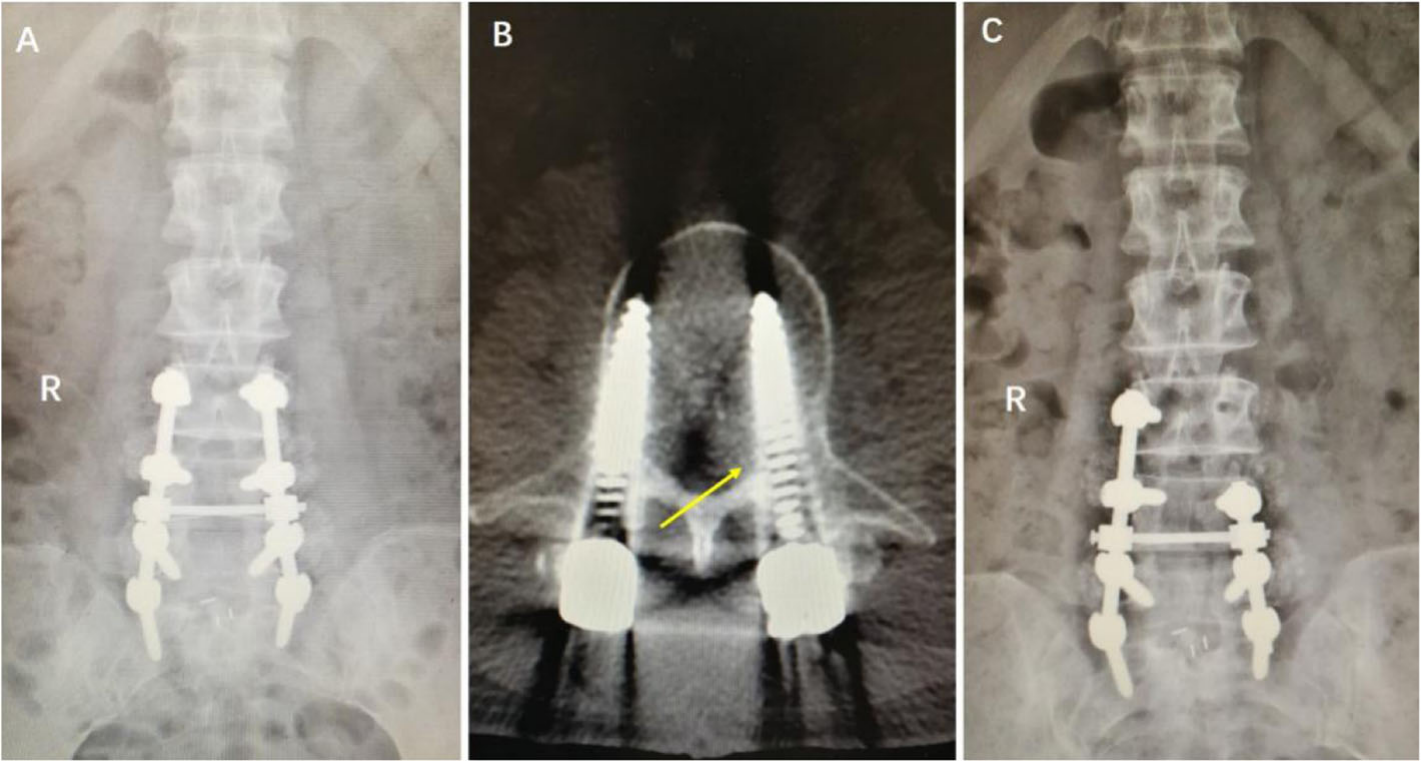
A 62-year-old female experienced pain in the front of her left thigh and had grade 1 hip flexion muscle strength 3 days after an L3–S1 PLIF. a Postoperative x-ray. b CT scan showed that the left L3 pedicle screw intruded the inner pedicle wall (arrow). c The left L3 pedicle screw was removed after reoperation. The patient’s symptoms were relieved after reoperation
